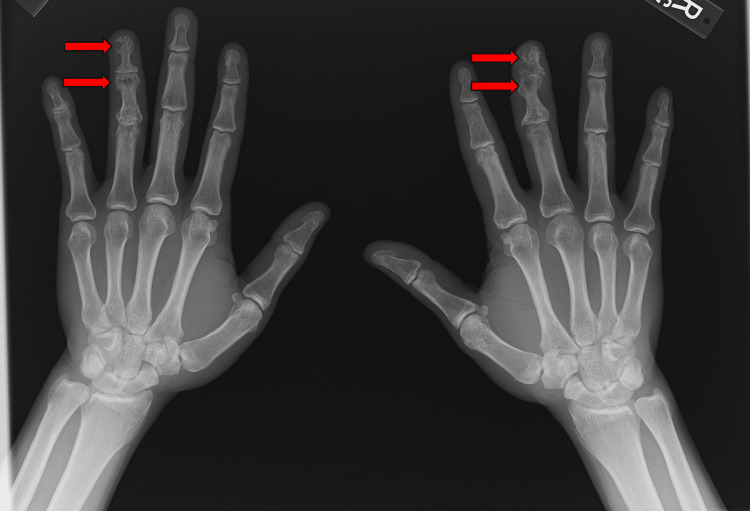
Bilateral hand X-ray showing osseous sarcoidosis affecting the right third digit and left fourth digit
